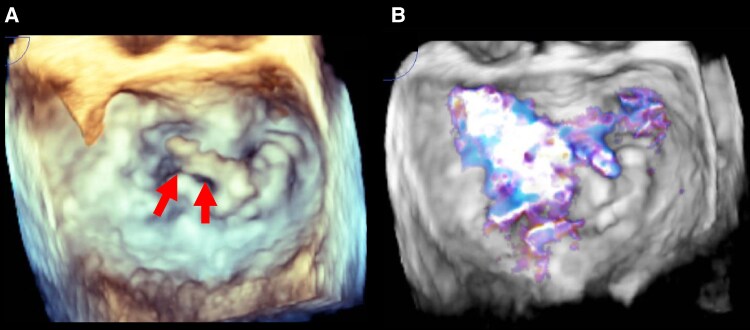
Three-dimensional transoesophageal echocardiography of a 73-year-old man. Transoesophageal echocardiography confirmed mitral leaflet prolapse resulting from chordal rupture of the middle scallop of the posterior mitral leaflet (*A*, arrows) concomitant with significant regurgitant jet (*B*).
